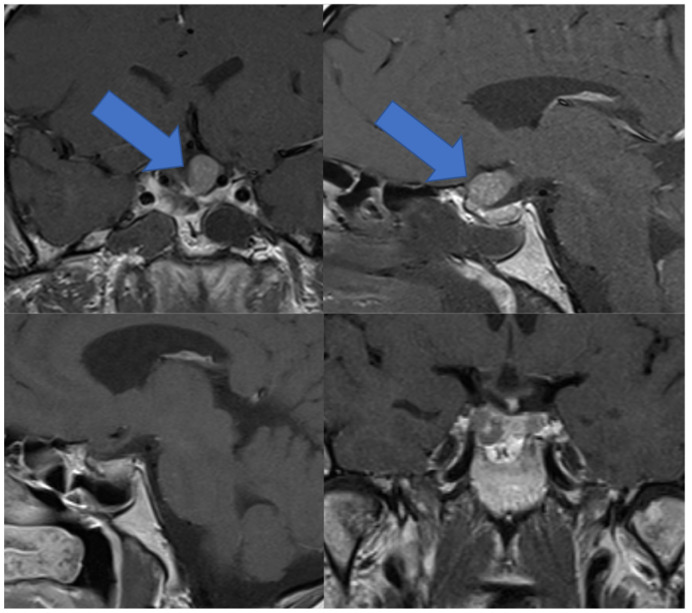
Intraoperative MRI depicting adenoma remnant and follow-up MRI 3 months after surgery showing decompressed chiasm and fat graft used for reconstruction of the sella.
